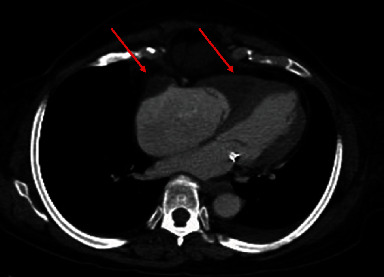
CTA showing evidence of right atrial and right ventricular apical thrombus (red arrows).
